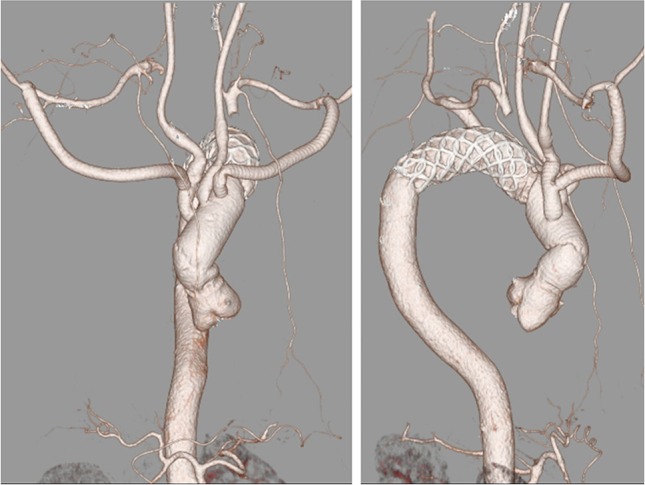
Postoperative computed tomography angiography.
